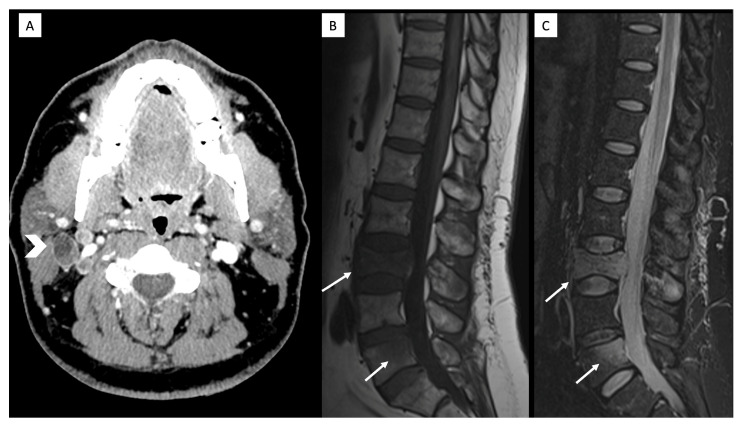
Neck CT and spinal MRI of Patient 3. Axial contrast-enhanced neck CT (A) shows multiple necrotic right cervical lymph nodes (arrowhead). Sagittal spinal MRI T1-weighted (B) and STIR (C) images show metastatic infiltration of L3 and L5 (arrows) and a pathological fracture of L3.
